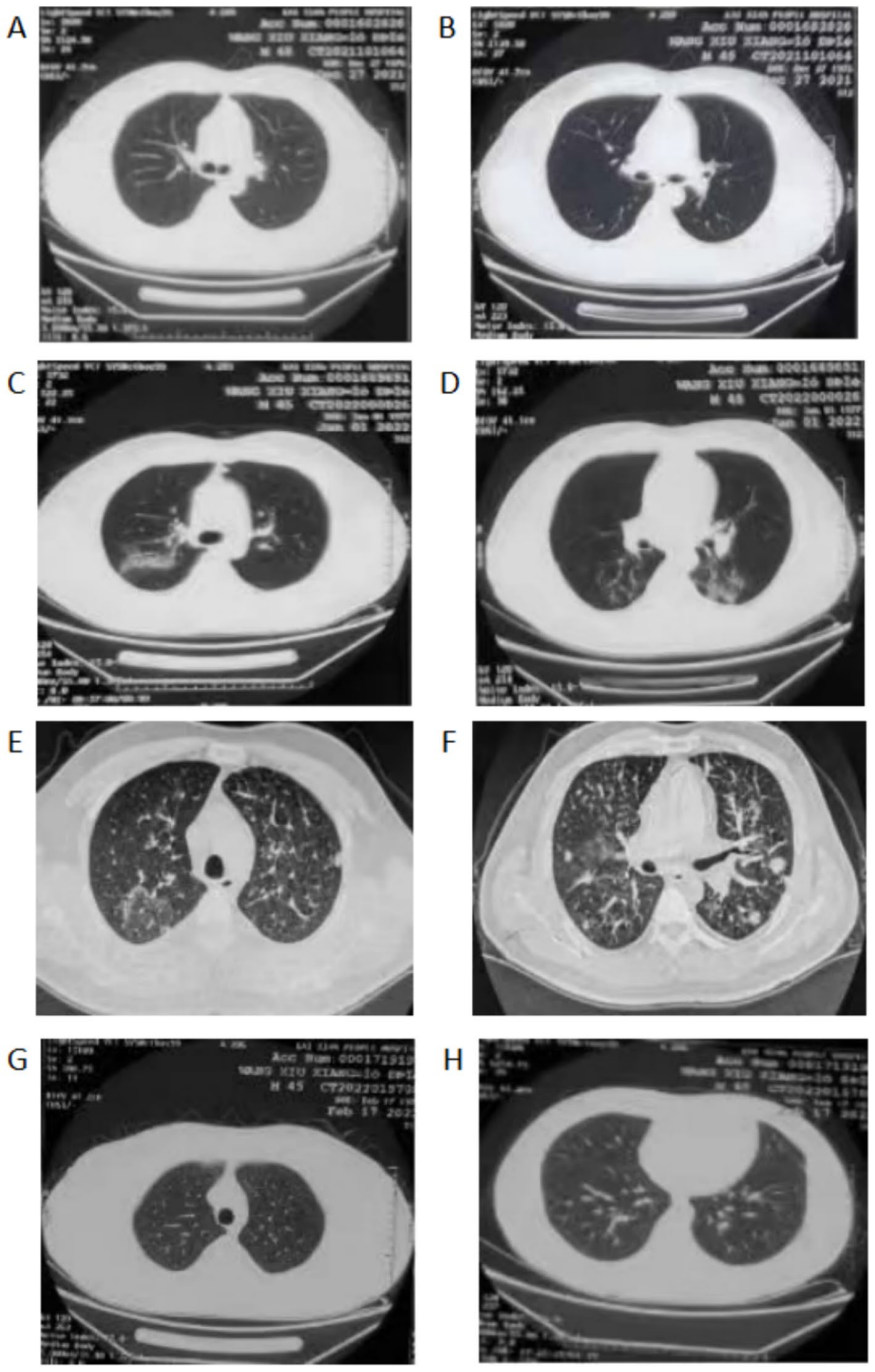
CT images of patients at different times [(A,B) show the lung CT of D-10, (C,D) show the lung CT of D-5, (E,F) show the lung CT of D 9, (G,H) show the lung CT of D 47].
